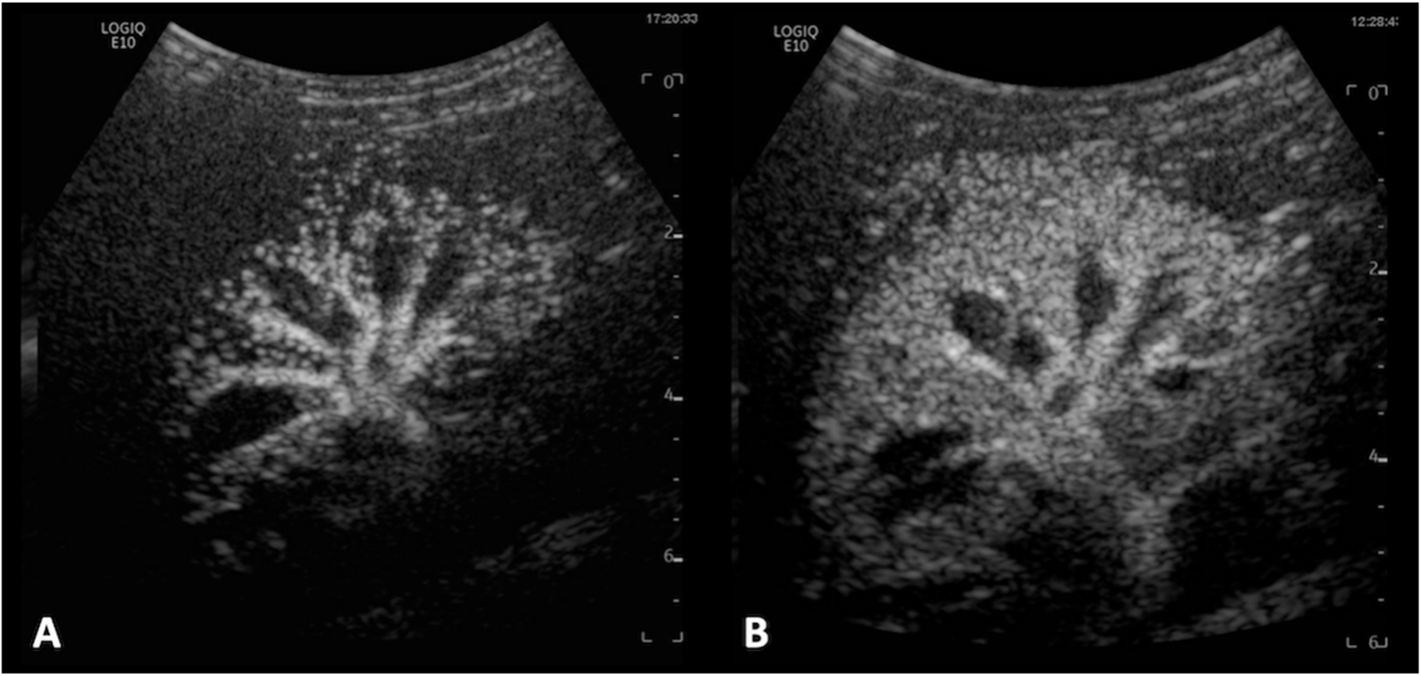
Contrast enhanced ultrasound. Single frames from a contrast enhanced US video loops taken immediately after sulfur hexafluoride lipid-type A microspheres are injected and flushed with 10mls saline. a Immediately pre right renal artery angioplasty with reduced cortical perfusion to the right kidney and b immediately post angioplasty with overall improved perfusion
